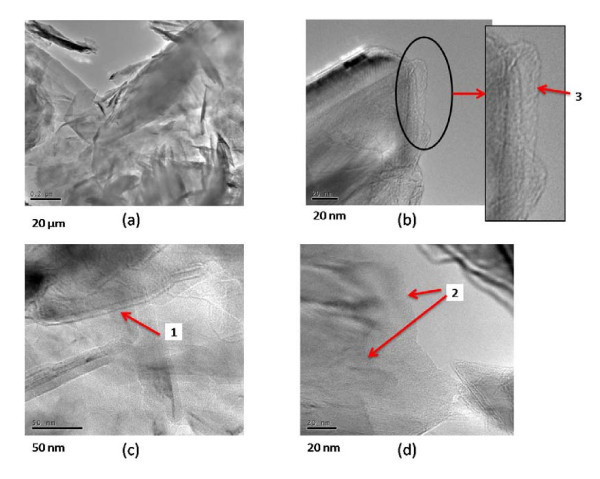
TEM images. TEM images with ultrasonic oscillation (a) FLG, (b) Edge of graphene sheet, (c) and (d) folded FLG.
